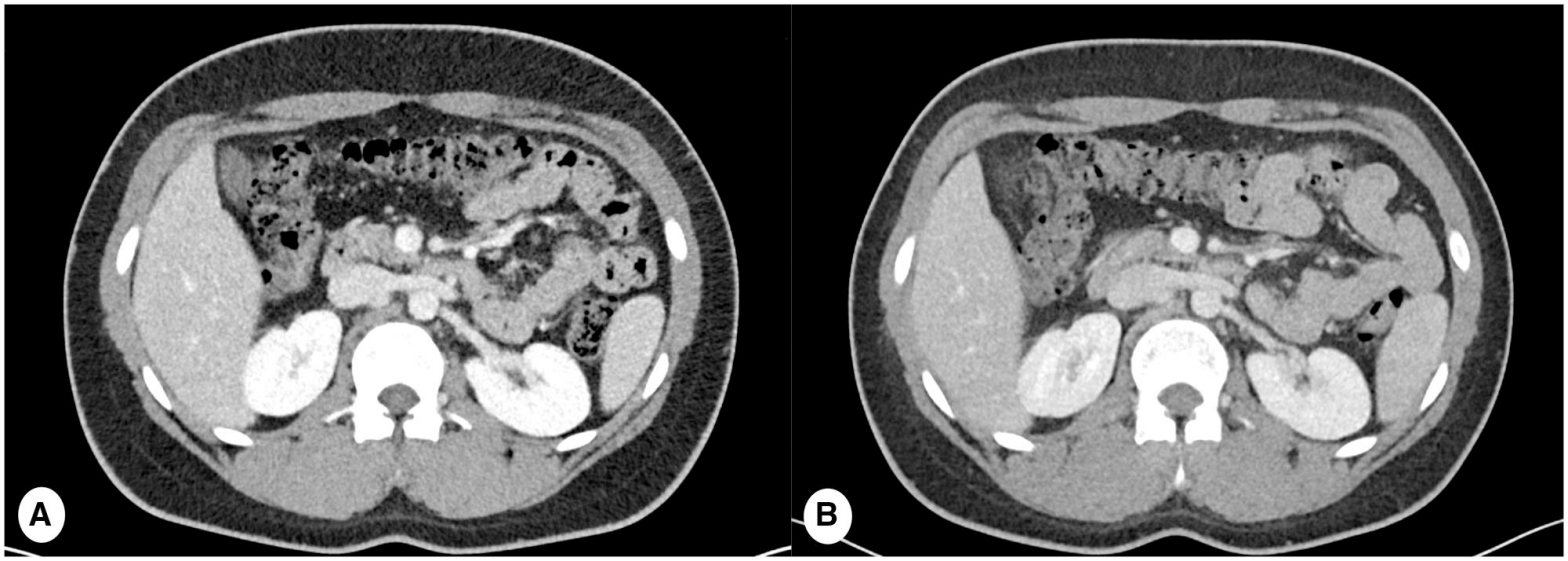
Comparison of abdominal imaging in the same patient scanned for Testicular seminoma. (A) PCD-CT scan and (B) EID-CT, both conducted with a uniform window width (WW) and window level (WL) set at 400 and 40, respectively. Both images had a slice thickness of 3 mm, reconstruction kernel Br40, IR Q3. The PCD-CT images exhibit a notably higher Signal-to-Noise Ratio (SNR) compared to the EID-CT image as it showcases enhanced contrast at the level of the kidneys. PCD-CT also provides sharper delineation of organ contours and blood vessels.
